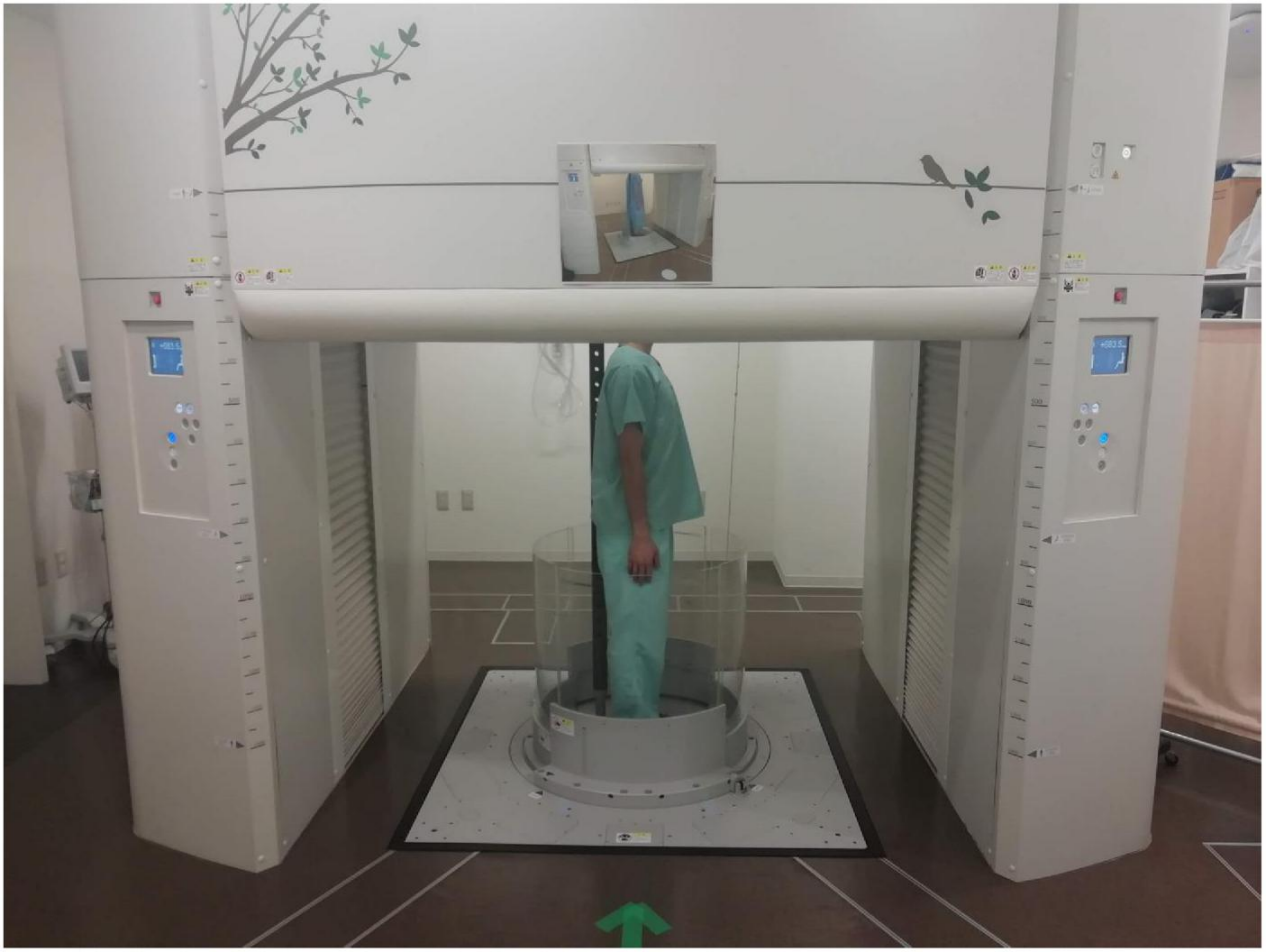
A 320-detector-row upright computed tomography (CT) scanner (prototype TSX-401R; Canon Medical Systems). Upright CT examinations were performed with the patient arms down.
